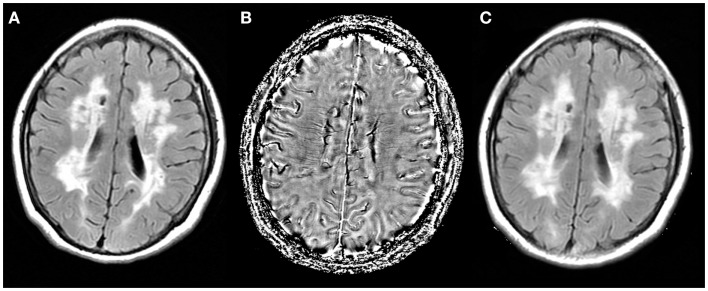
Axial T2 fluid attenuated inversion recovery (FLAIR) images were coregistered to the phase images. (A) T2 FLAIR image; (B) phase image of the same patient was set as a reference; (C) coregistered T2 FLAIR image.
